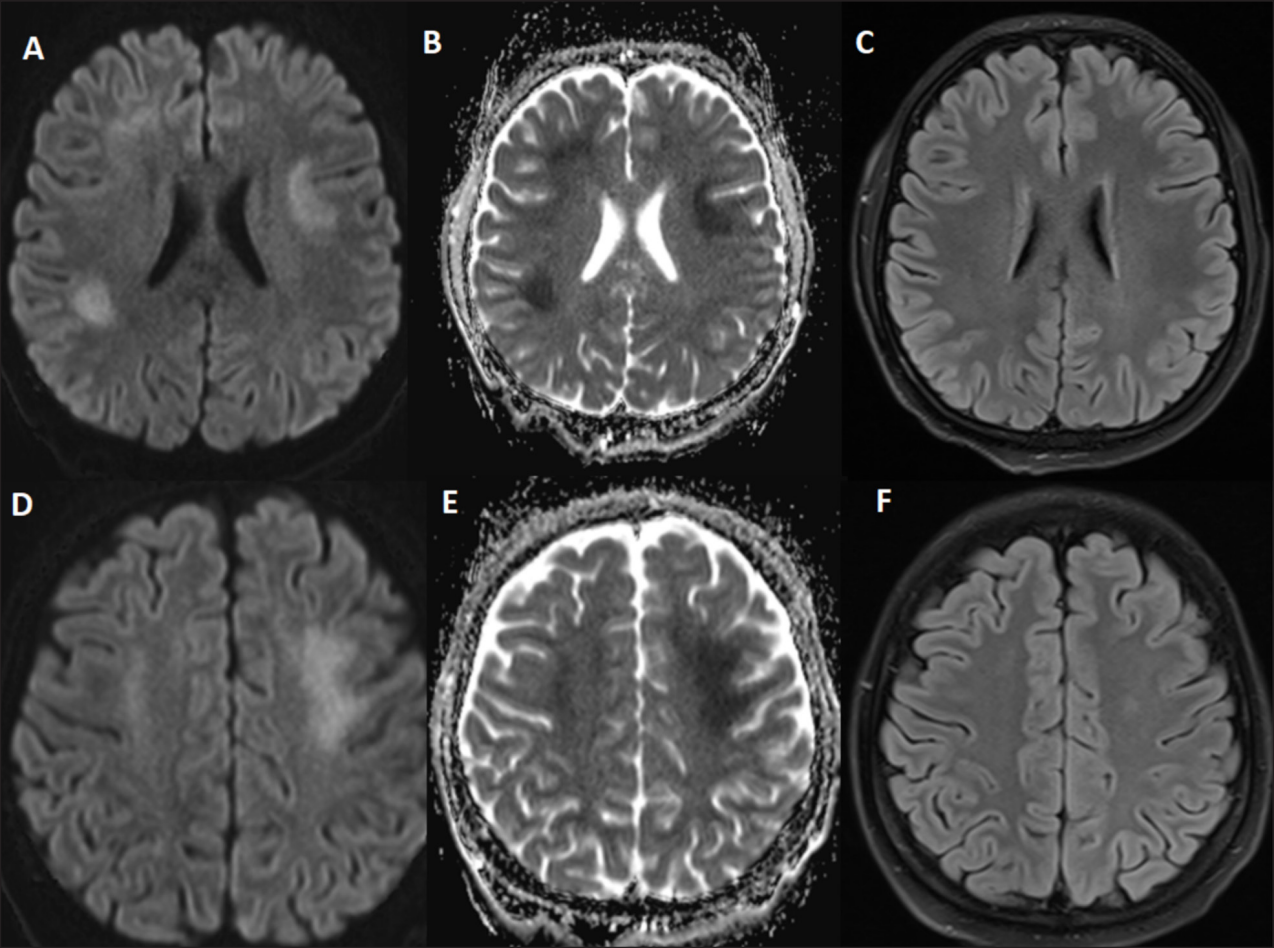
Brain MRI showing bilateral hyperintense diffusion-weighted imaging signals (A, D) and decreased apparent diffusion coefficient (B, E) in the white matter of the frontal and parietal lobes. Axial fluid attenuated inversion recovery image was normal (C, F).
